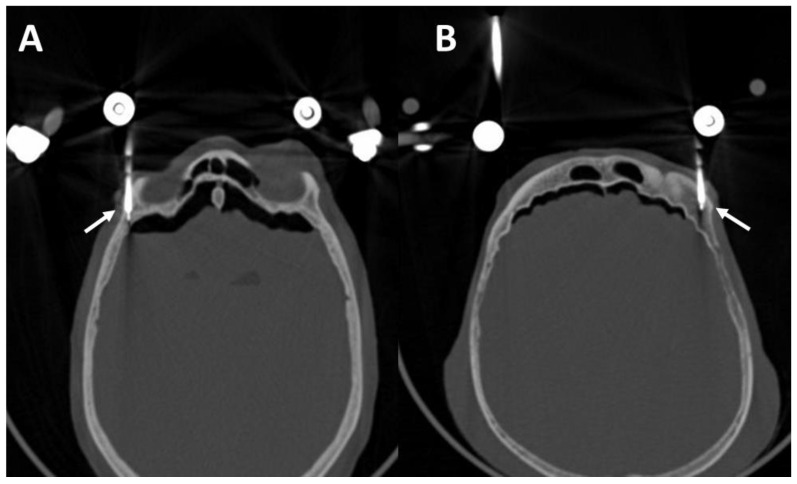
CT scans of the fractured skull with right (A) and left and right (B) supraorbital pins (white arrows).
